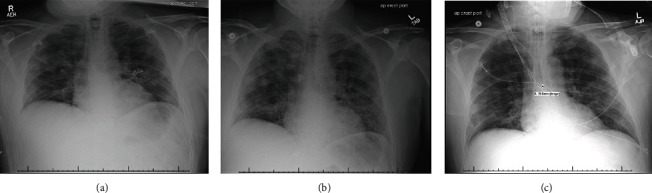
(a) Early chest X-ray (CXR) on day of admission consistent with patchy airspace infiltrates concerning for early viral pneumonia. (b) Two days into admission, CXR shows diffuse ground glass and interstitial opacities with worsening airspace infiltrates. (c) Decrease in diffuse airspace infiltrates and ground glass opacities, six days after intubation.
